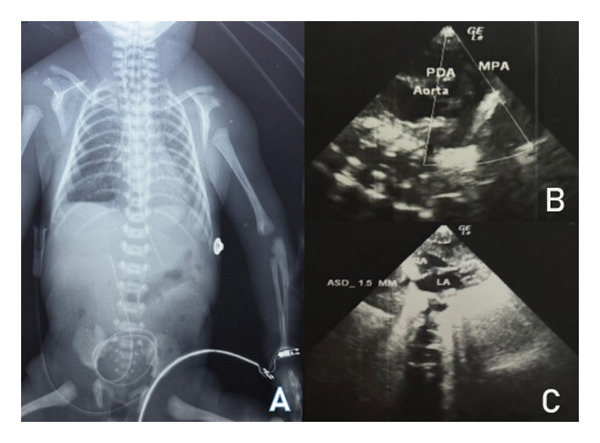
(A) Chest and abdomen x‐ray in AP view; (B) echocardiography finding showing patent ductus arteriosus; and (C) echocardiography finding showing atrial septal defect.
